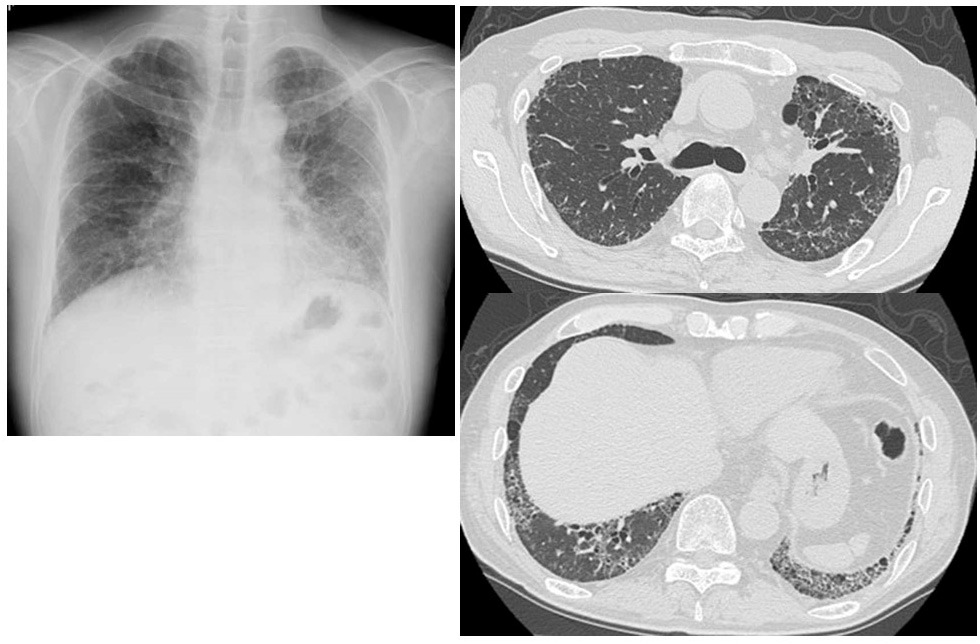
Chest radiograph and CT scans of Case 2 showing reticular opacities, traction bronchiectasis, and some ground-glass attenuation in the bilateral lung fields.
